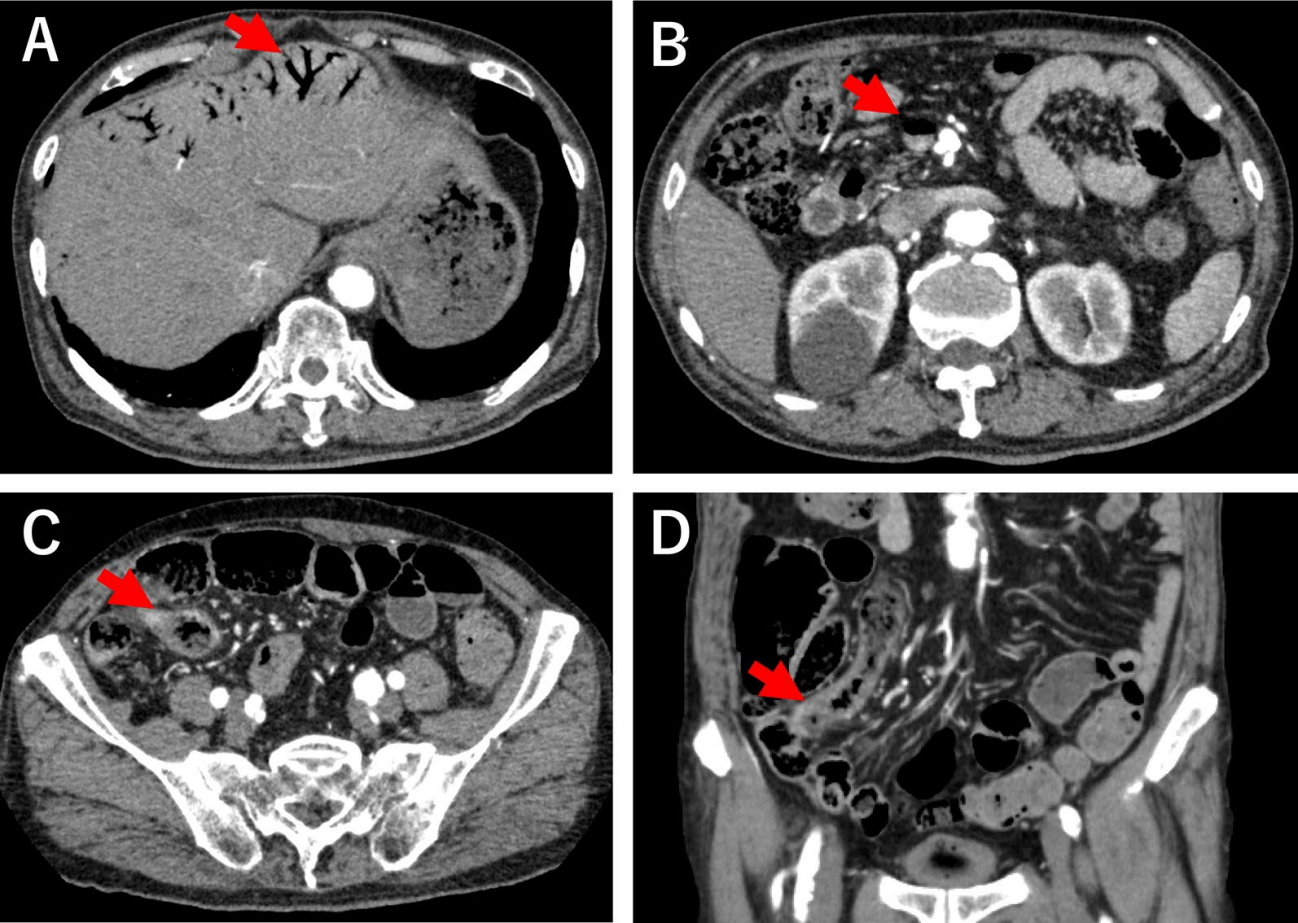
Contrast-enhanced CT. A Contrast-enhanced CT of the abdomen revealed intrahepatic portal vein gas (arrows). B CT showing superior mesenteric vein gas (arrow). C, D Terminal ileum is edematous and shows thickening of the wall; however, no obvious contrast effect loss is visible (arrow)
